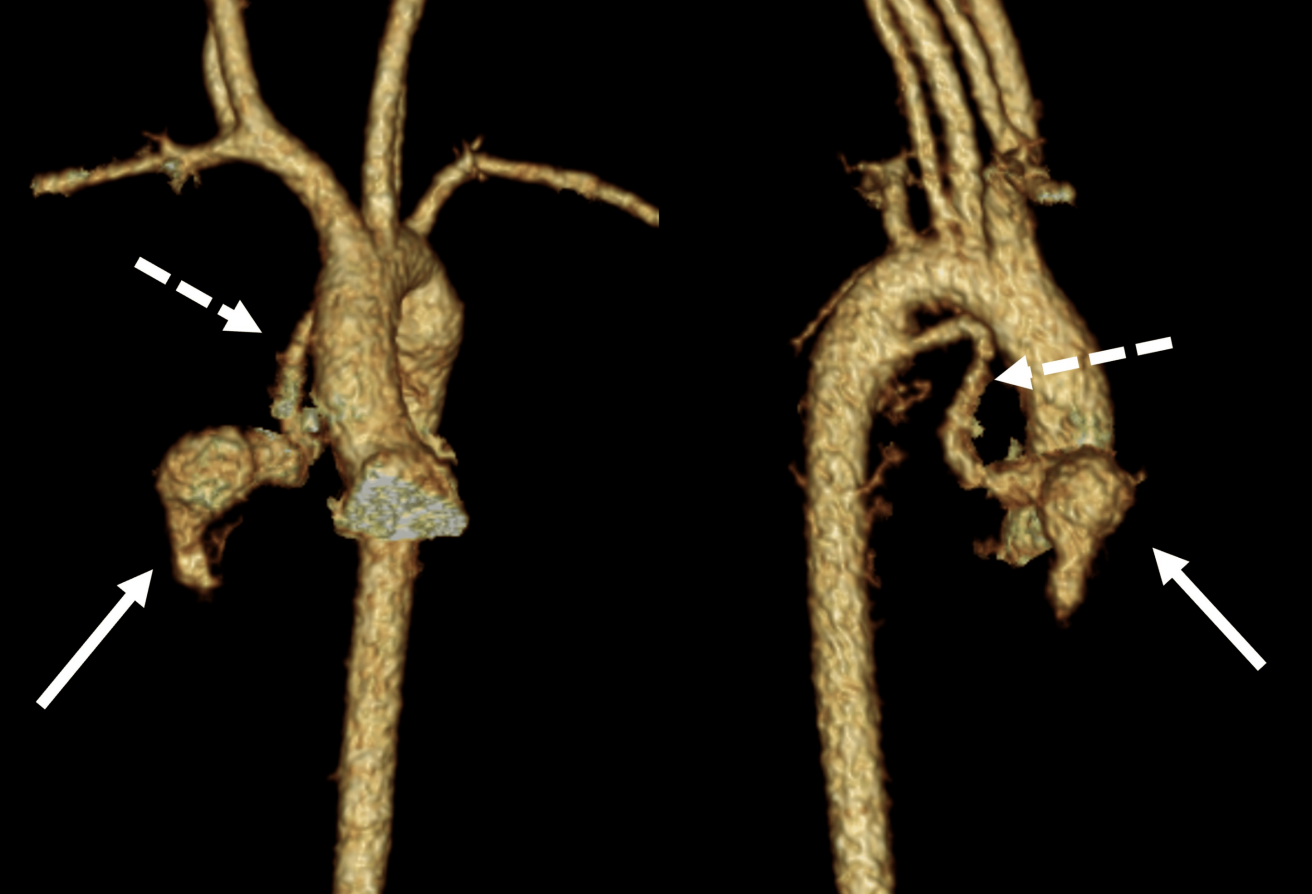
Contrast-enhanced computed tomography (three-dimensional reconstructed image) Contrast-enhanced computed tomography (three-dimensional reconstructed image) demonstrated an abnormal vessel branching off the descending aorta with an associated aneurysm. The arrow indicates an aneurysm and the dashed arrow indicates an abnormal vessel.
